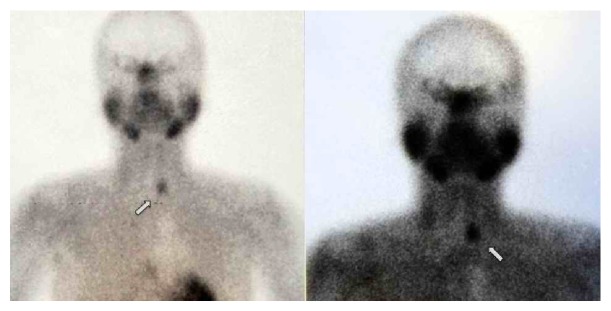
Abdominal CT shows radiopaque material filling the gallbladder and ultrasound shows an acoustic shadow consistent with gallstones (white arrows).
